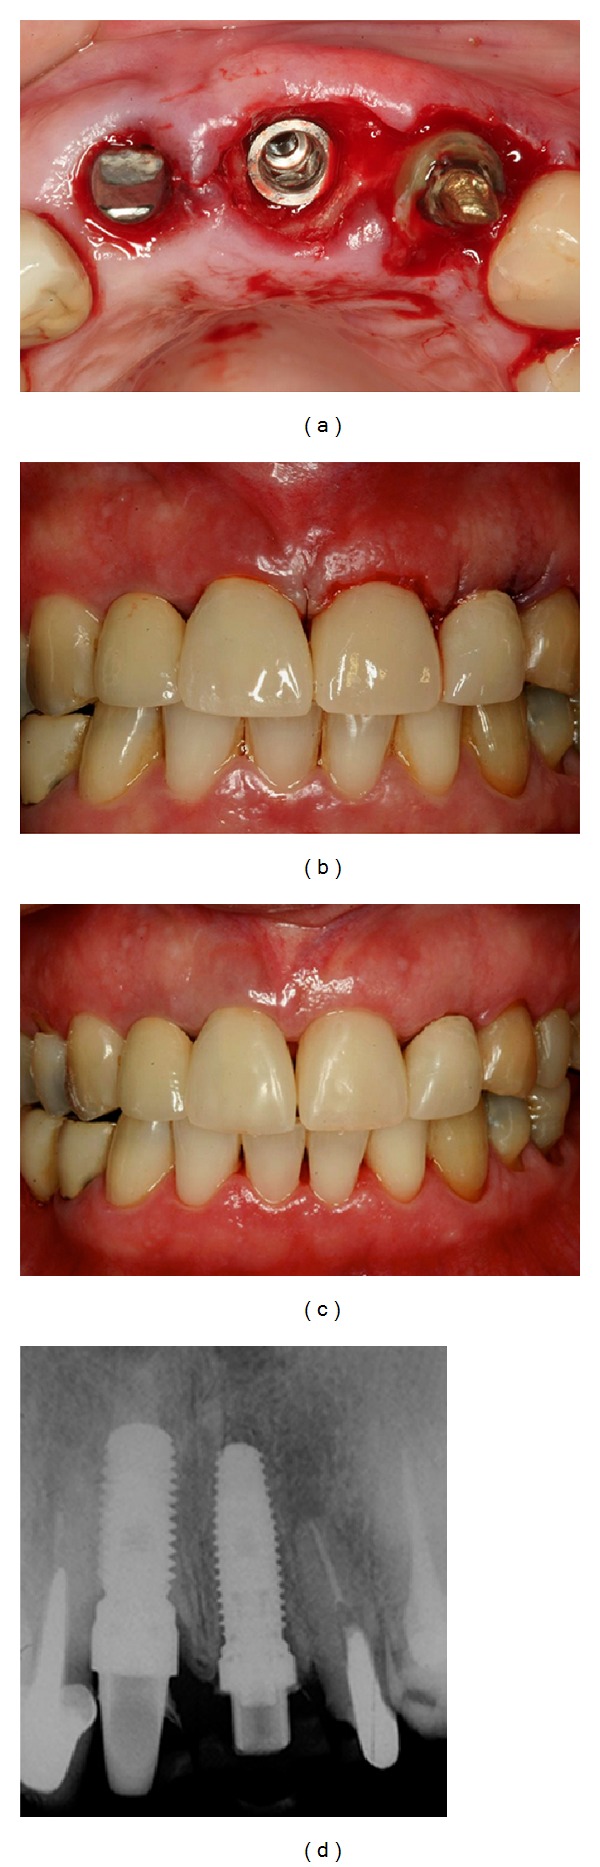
(a) Occlusal view of the implant placed. (b, c) Immediate postoperative views with provisional resin crown in the central and lateral incisor teeth. (d) Periapical radiography after implant installation.
